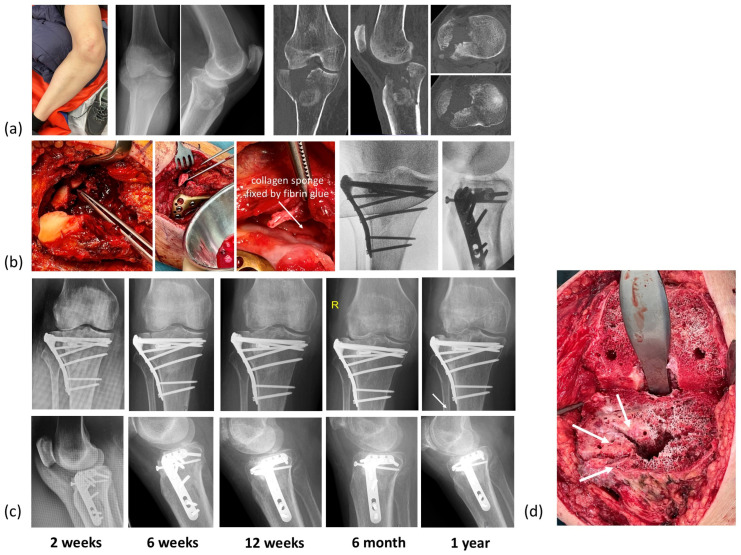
Comminuted tibia plateau fracture with valgus deformity, significant bone loss, and frontal instability in a 64-year-old female. (a) Clinical picture and radiographs/CT scan. (b) The intraoperative procedure included restoration of the joint line using an allograft block (50% of a femoral head) and bioactivated TCP granules. The cartilage defect zone was covered by a fibrin glue fixed collagen sponge to obtain a barrier between bone substitute materials and the intraarticular joint space. The fracture was stabilized by a tibia plateau plate and locking screws (Fa. Synthes, USA). (c) The postoperative follow-up over 1 year showed progressive healing of the fracture with a solid bone stock in case of later joint replacement. A small defined subchondral defect zone remains (arrow). (d) Due to post-traumatic osteoarthritis the patient underwent total knee replacement 1.5 years after ORIF. The intraoperative situs showed solid bone formation within the former defect zone (arrows).
